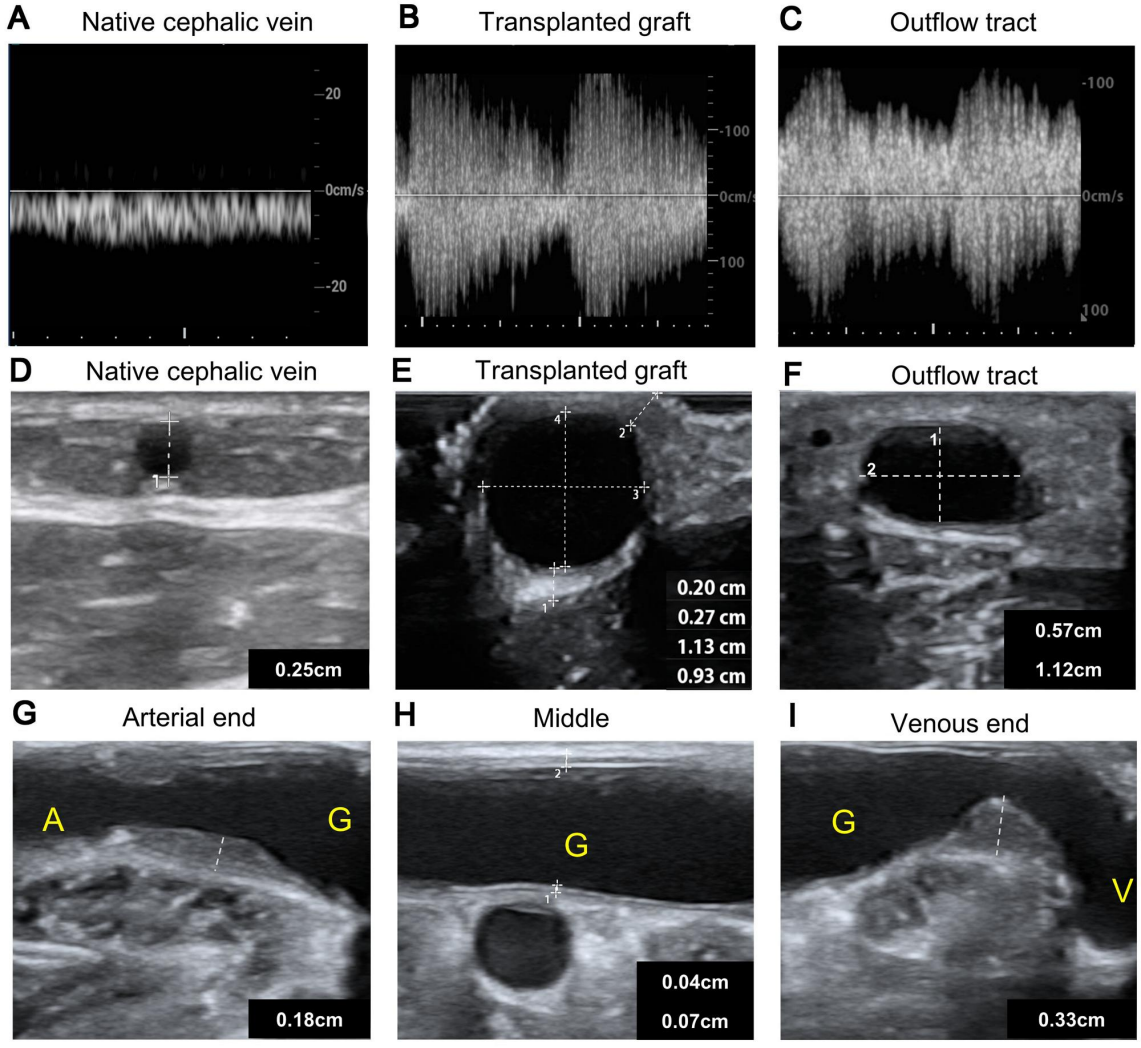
The non-invasive ultrasound evaluation of the decellularized vascular graft in human. (A–C) The ultrasound spectra of the cephalic vein (A), decellularized graft (B) and outflow tract of decellularized graft (C). (D–F) The vascular diameters of the cephalic vein (D), transplanted graft (E) and outflow tract of decellularized graft (F). (G–I) the extent of intimal hyperplasia at the arterial anastomotic end (G), Middle segment (H) and venous anastomotic end (I) of the decellularized graft. A represents the artery connected to the graft; G represents the graft; ‘V’ represents the vein outflow tract connected to the graft.
